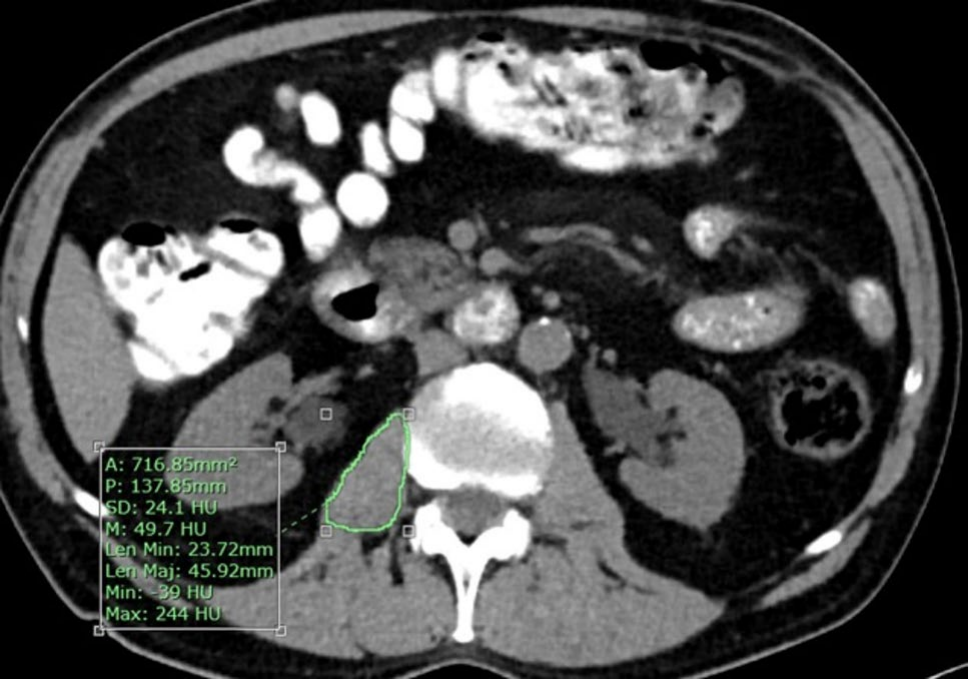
Axial image from a non-contrast preoperative computed tomography scan of a 77-year-old patient. The area of the right psoas muscle, measured at the level of L3, is 717 mm^2^ with an average density of 49.7 Hounsfield units
